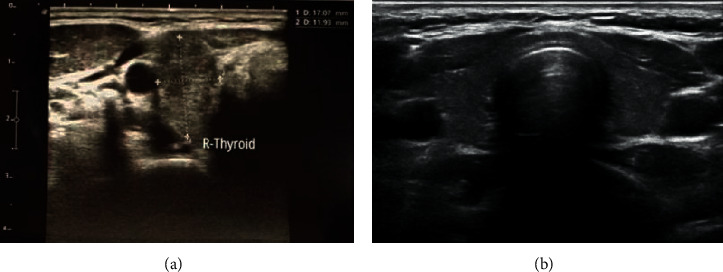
(a) A neck ultrasound showed a solitary 1 cm right thyroid nodule. (b) Follow-up neck ultrasound revealed no nodularity.
